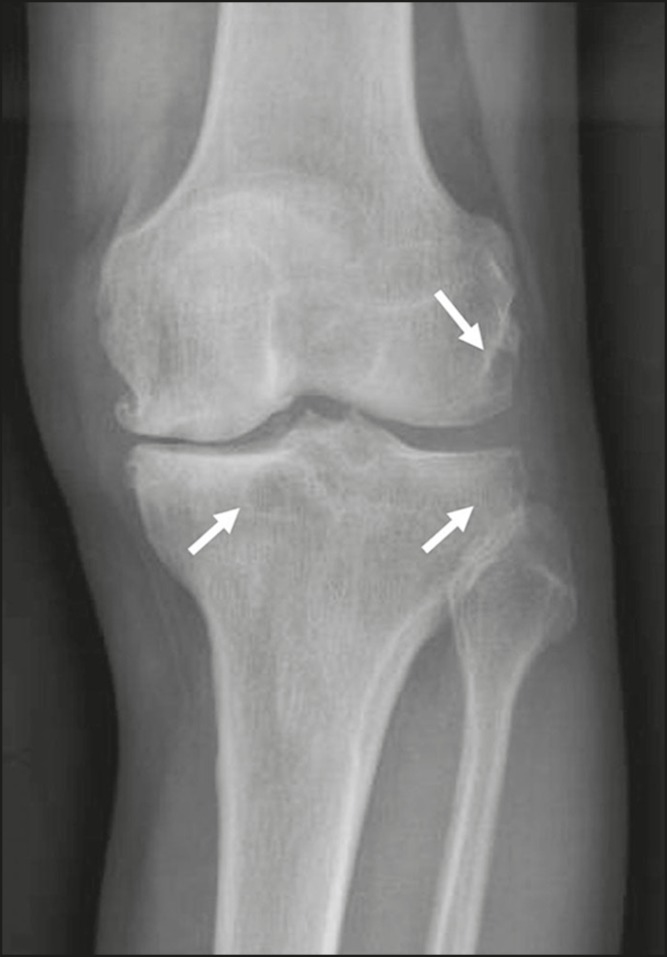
A 73-year-old male with knee pain. Anteroposterior X-ray of the left
